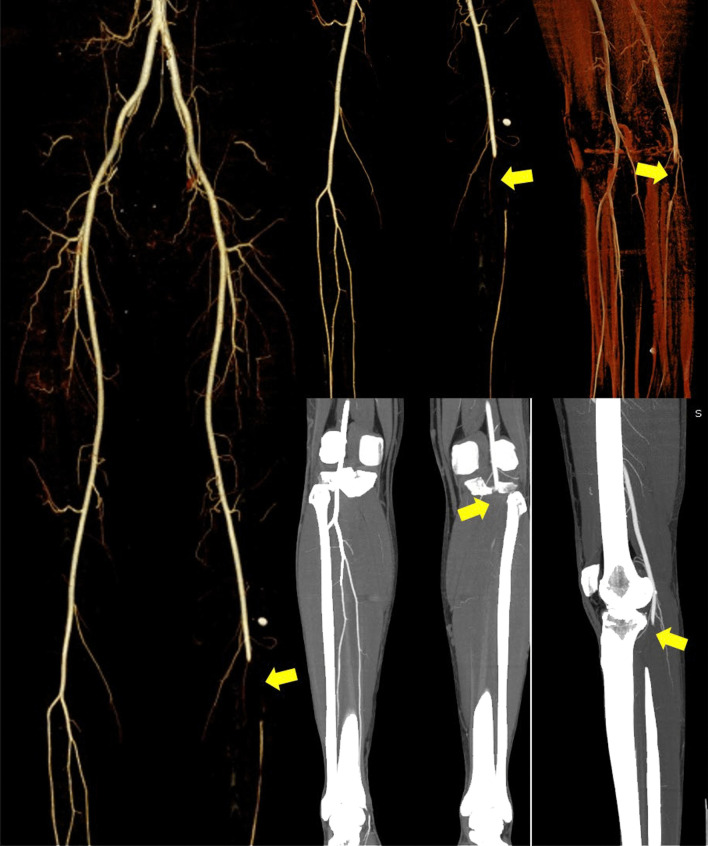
A 21-year-old, normal weight and non-smoker man presented to the emergency department with new onset of cool and painful left leg, ten days after having the second dose of COVID-19 vaccine from AstraZeneca. Volume Rendering and Maximum Intensity Projection CT angiography images show acute segmental thrombotic occlusion of the popliteal artery (yellow arrows). He had no underlying illnesses, trauma, surgery, infection, or immobilization. There was no known thrombophilia. Unfortunately, the patient underwent critical ischemia and urgent limb amputation
